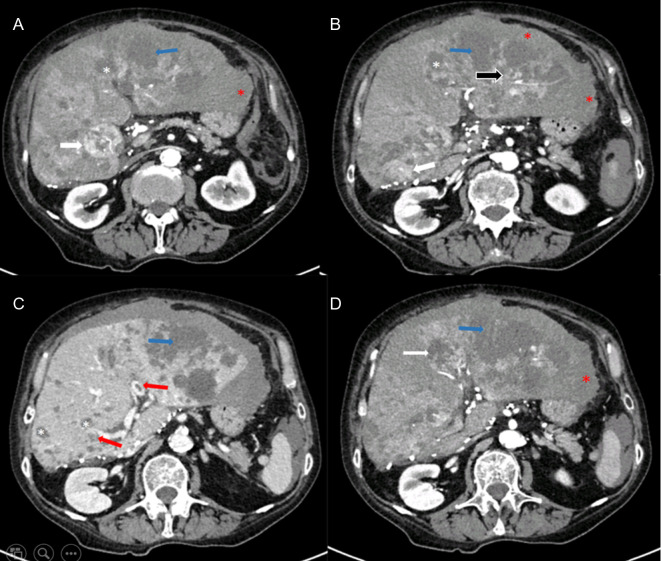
Abdominal axial CT images of the liver in arterial (A and B) and venous phase (C and D) showing multiple liver lesions of various characteristics (white arrow-HCC; black arrow- ruptured HCC; white asterisk-simple liver cysts; blue arrow-abscess; red asterisk-hematoma; red arrow-portal vein thrombosis).
